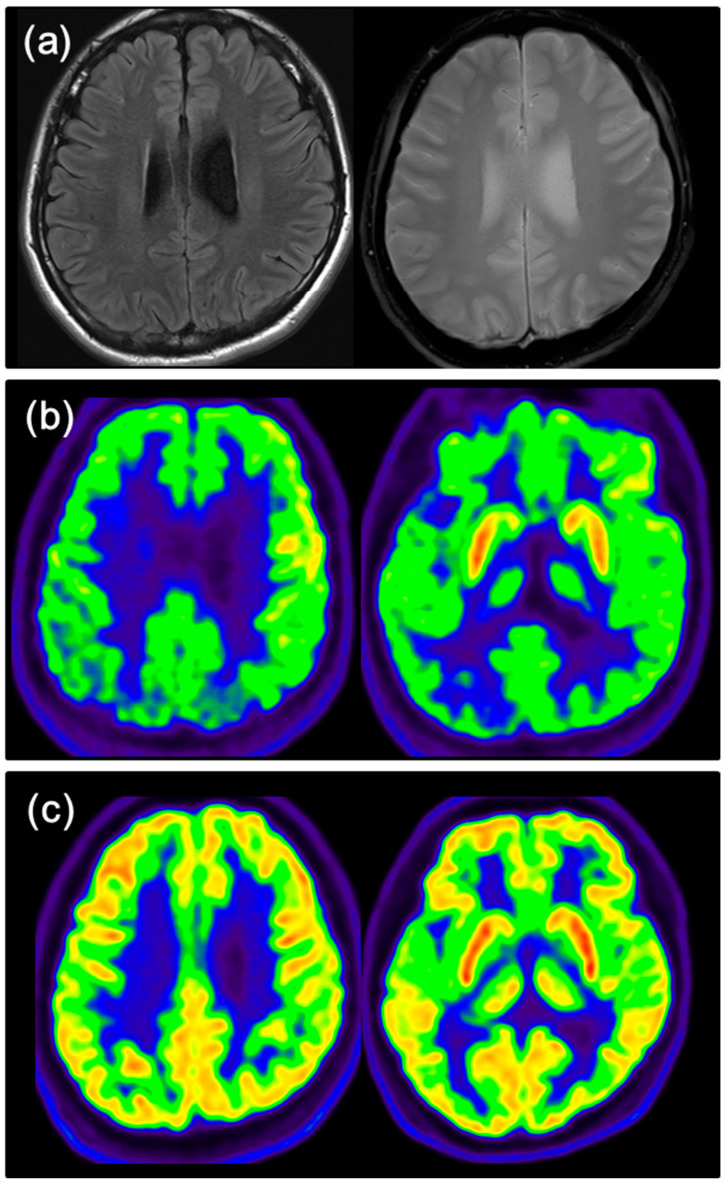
Magnetic resonance imaging depicted no abnormality in the FLAIR and GRE sequences during the acute phase of anti-NMDA receptor encephalitis (a). Brain 18F-fluorodeoxyglucose positron emission tomography showed a pronounced typical anteroposterior gradient pattern with marked hypometabolism in bilateral parietooccipito-temporal lobes and preserved metabolism in basal ganglia during the acute phase (b), which improved after a 4-month follow-up (c).
